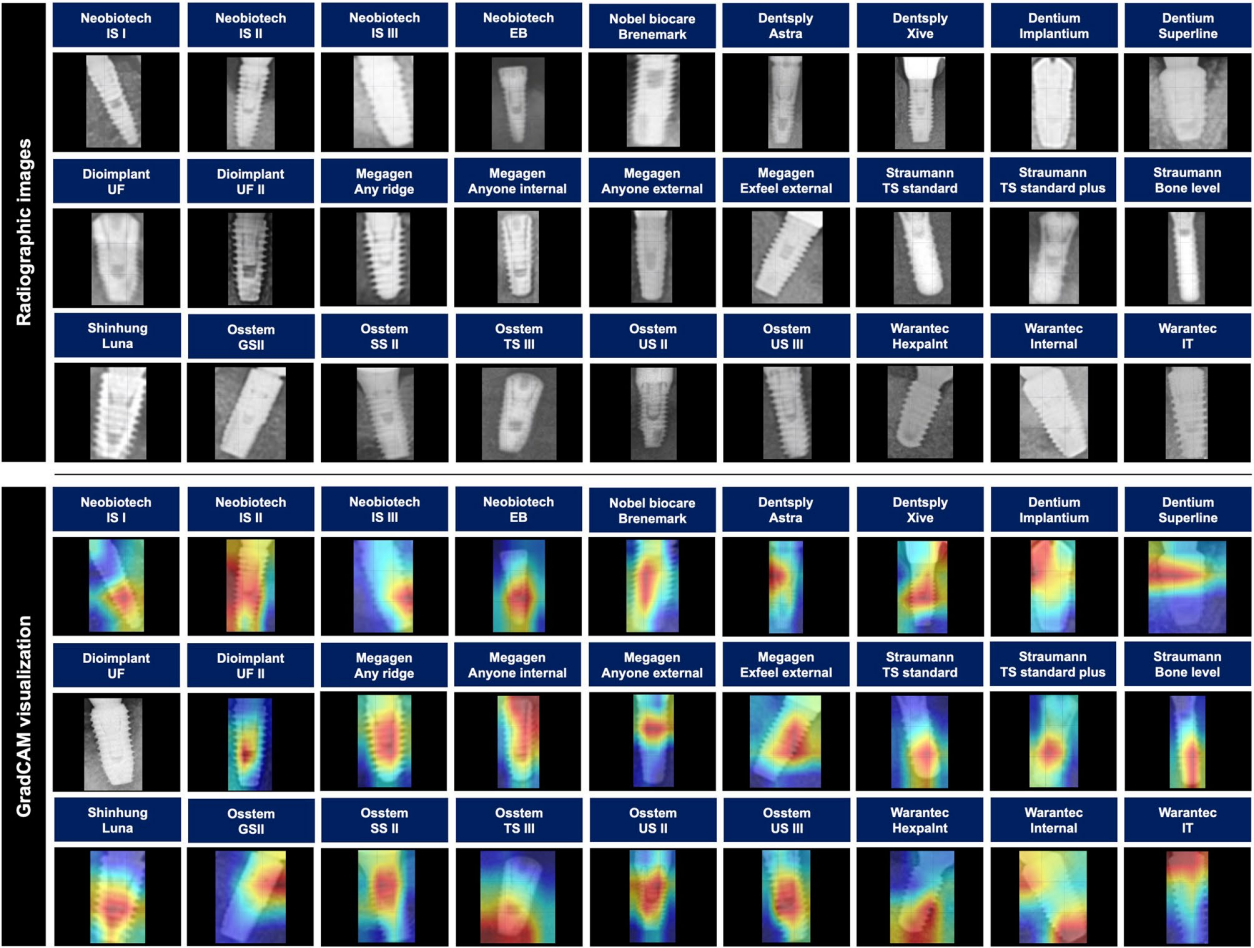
Dataset containing 116,756 panoramic and 40,209 periapical radiographic images and comprising 10 manufacturers and 27 types of dental implant systems.
